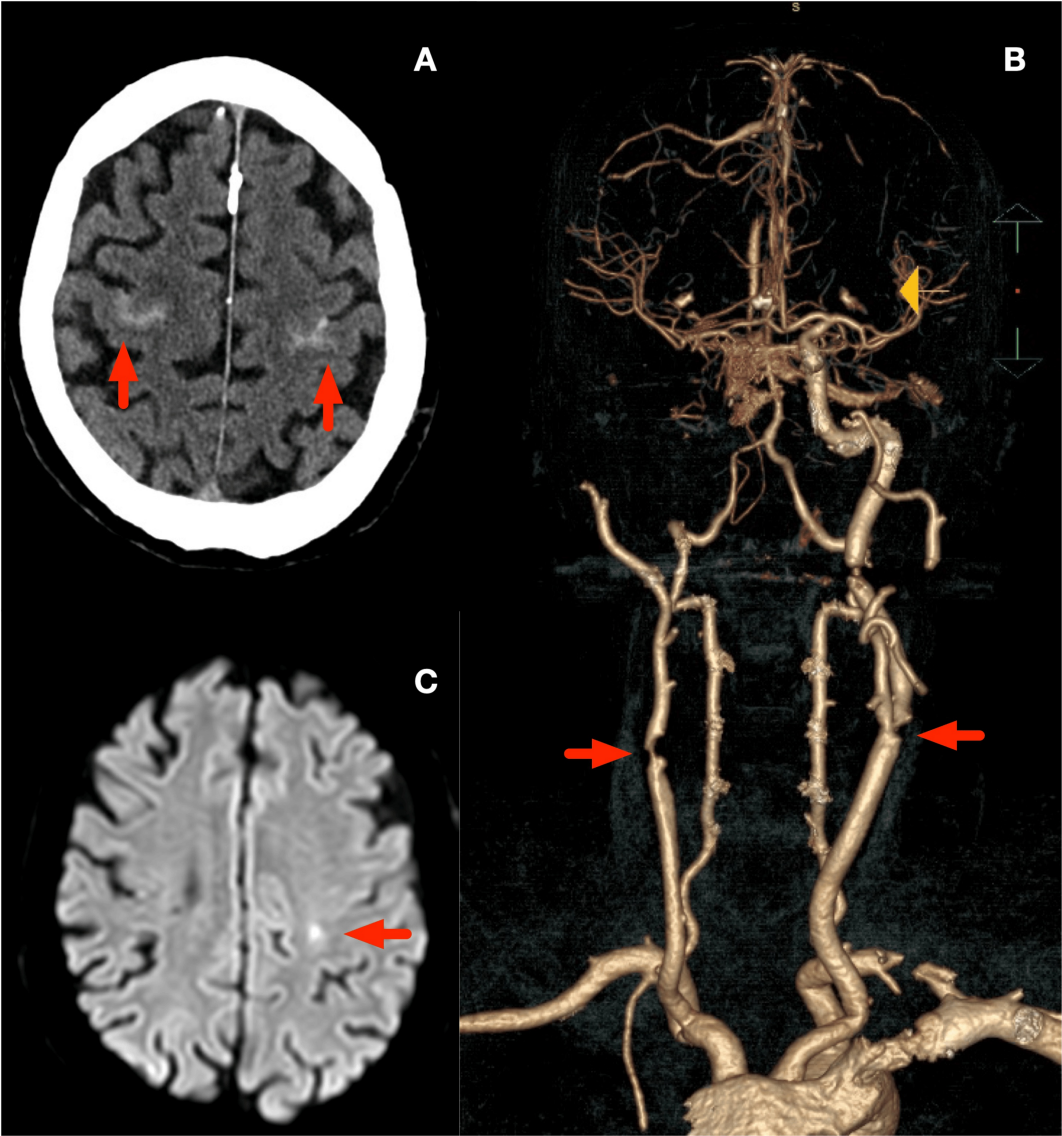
Neuroradiological findings. A: Non-contrast CT scan (NCCT) showing bilateral convexity subarachnoid haemorrhage (cSAH) (red arrows). B: Angio-CT volume rendering reconstruction, showing right internal carotid artery (ICA) steno-occlusion and left ICA stenosis (red arrows). C: Diffusion-weighted magnetic resonance (DWI-MR) showing left corona radiata acute ischemic lesion (red arrow).
